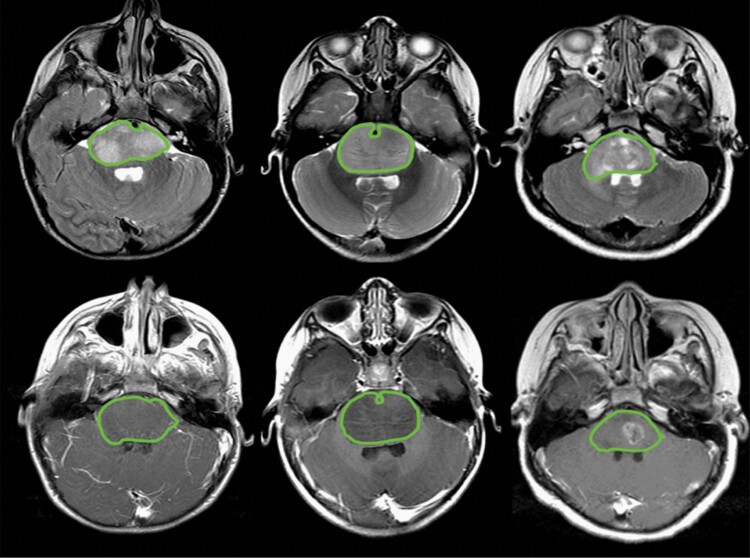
Examples of tumor regions of interest over the tumor. Tumor segmentation was performed over abnormal signals on T2-weighted MRI (top) and corresponding gadolinium-enhanced T1-weighted MRI (bottom) to generate tumor volume.
